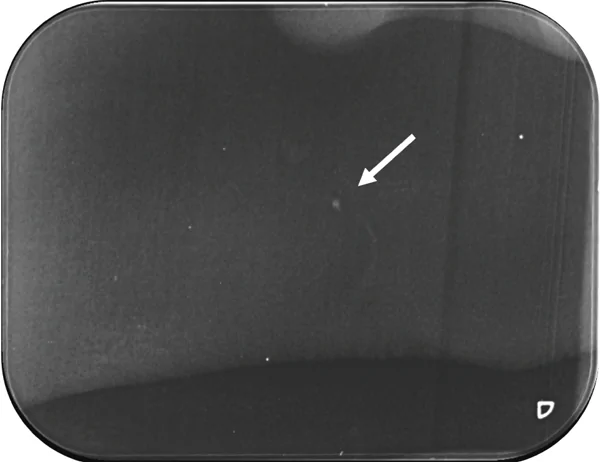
Radiographic findings at the initial visit. Dental radiograph showing a radio‐opaque entity within the soft tissue of the upper lip. An irregularly shaped radio‐opaque object is observed (arrow).
